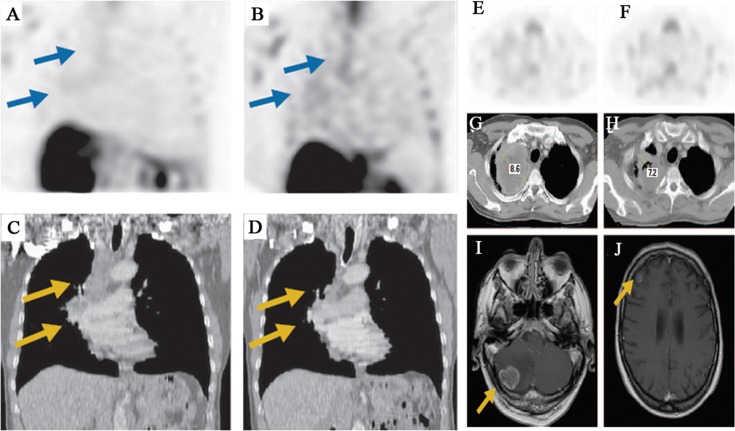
^99^mTc-Annexin V SPECT imaging of tumor response during platinum-based chemotherapy in advanced lung cancer A. Baseline and B. follow-up ^99m^Tc-Annexin V imaging demonstrate chemotherapy-induced increase of tumor tracer uptake (arrows). C. Baseline computed tomography demonstrates solid mass in the right upper lobe enlarged mediastinal lymph nodes (arrows). D. Follow-up computed tomography scan 8 weeks after the start of chemotherapy shows complete response (arrows). E. Baseline and F. follow-up ^99m^Tc-Annexin V imaging demonstrate therapy-induced decrease of Annexin V uptake. G. Baseline computed tomography shows heterogeneous mass in the right upper lobe. Follow-up H. computed tomography and magnetic resonance scans obtained 4 weeks later demonstrate local stable disease and brain metastases (arrows) in the I. cerebellum and J. right frontoparietal area.
